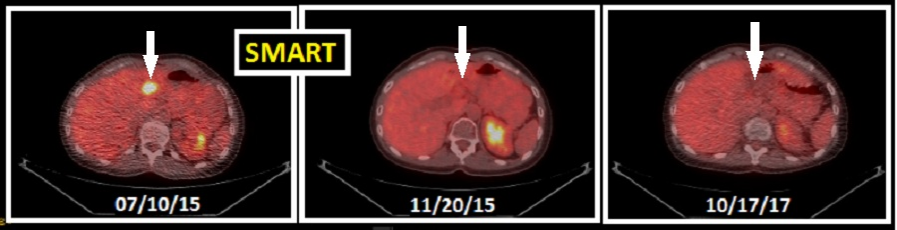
PET-CT pre- and post-treatment with stereotactic magnetic resonance-guided adaptive radiation therapy. Pre-therapeutic imaging on 07/10/15 shows a hypermetabolic activity adjacent to segment V/IVB of the liver which was absent on early evaluation after treatment on 11/20/15. The response was maintained till then as shown on her last imaging on 10/17/17. PET-CT: Positron emission tomography-computed tomography.
